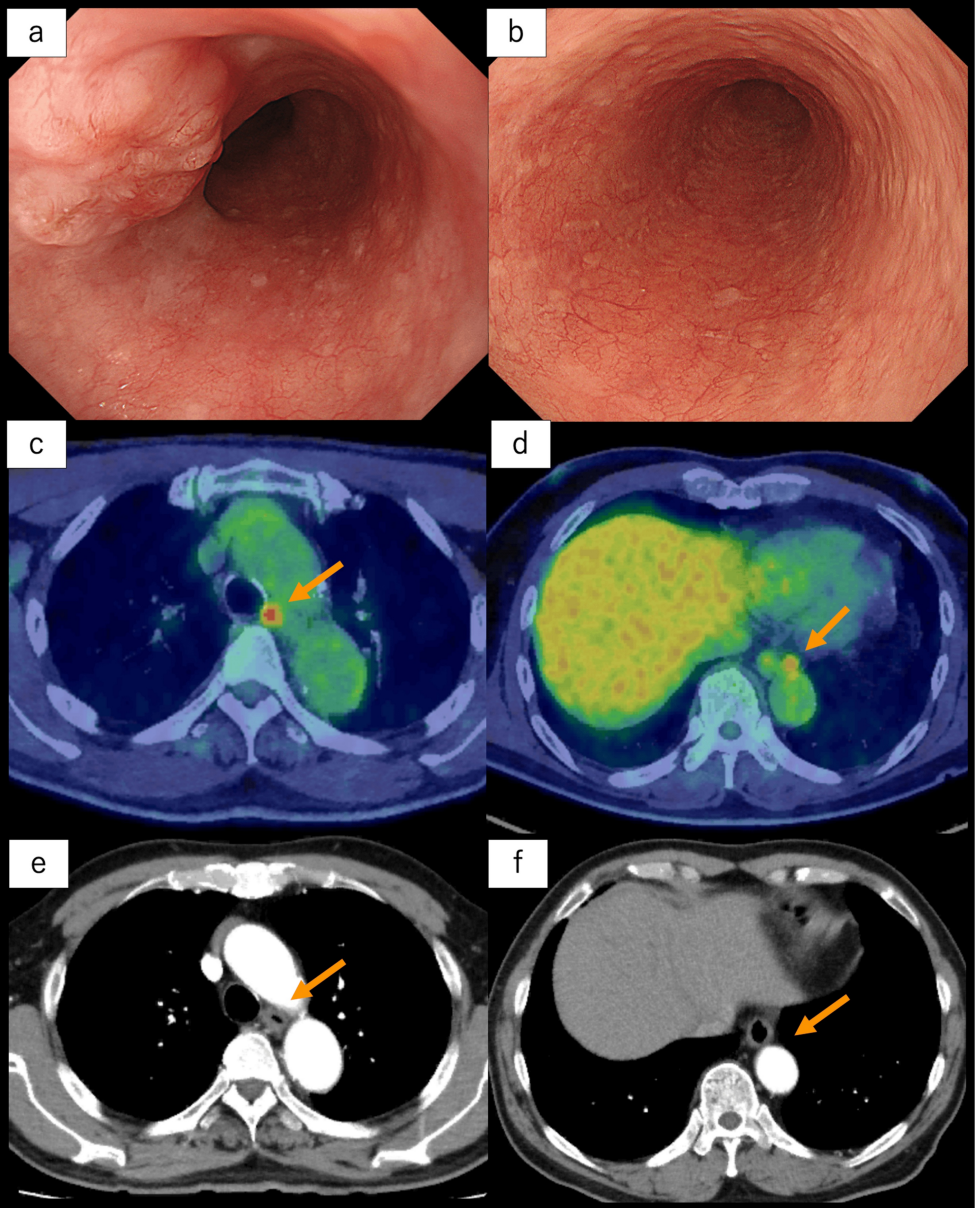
Imaging findings of esophageal primary lesion and metastatic lesions. (a) Endoscopic image showing a 0-IIc lesion, 25 mm in length, in the middle thoracic esophagus, 30 cm from the incisors. A biopsy confirmed the presence of squamous cell carcinoma. (b) Endoscopic image after two courses of neoadjuvant chemotherapy showing complete regression of the esophageal lesion. (c-d) Positron emission tomography-computed tomography scan findings (orange arrows). The images show two lymph node metastases detected at No. 106tbL (SUVmax: 5.43) (c) and No. 112aoA (SUVmax: 4.41) lymph nodes (d). (e-f) A contrast-enhanced computed tomography scan performed after neoadjuvant chemotherapy showed complete remission of enlarged lymph nodes (orange arrows). SUVmax: maximum standardized uptake value.
